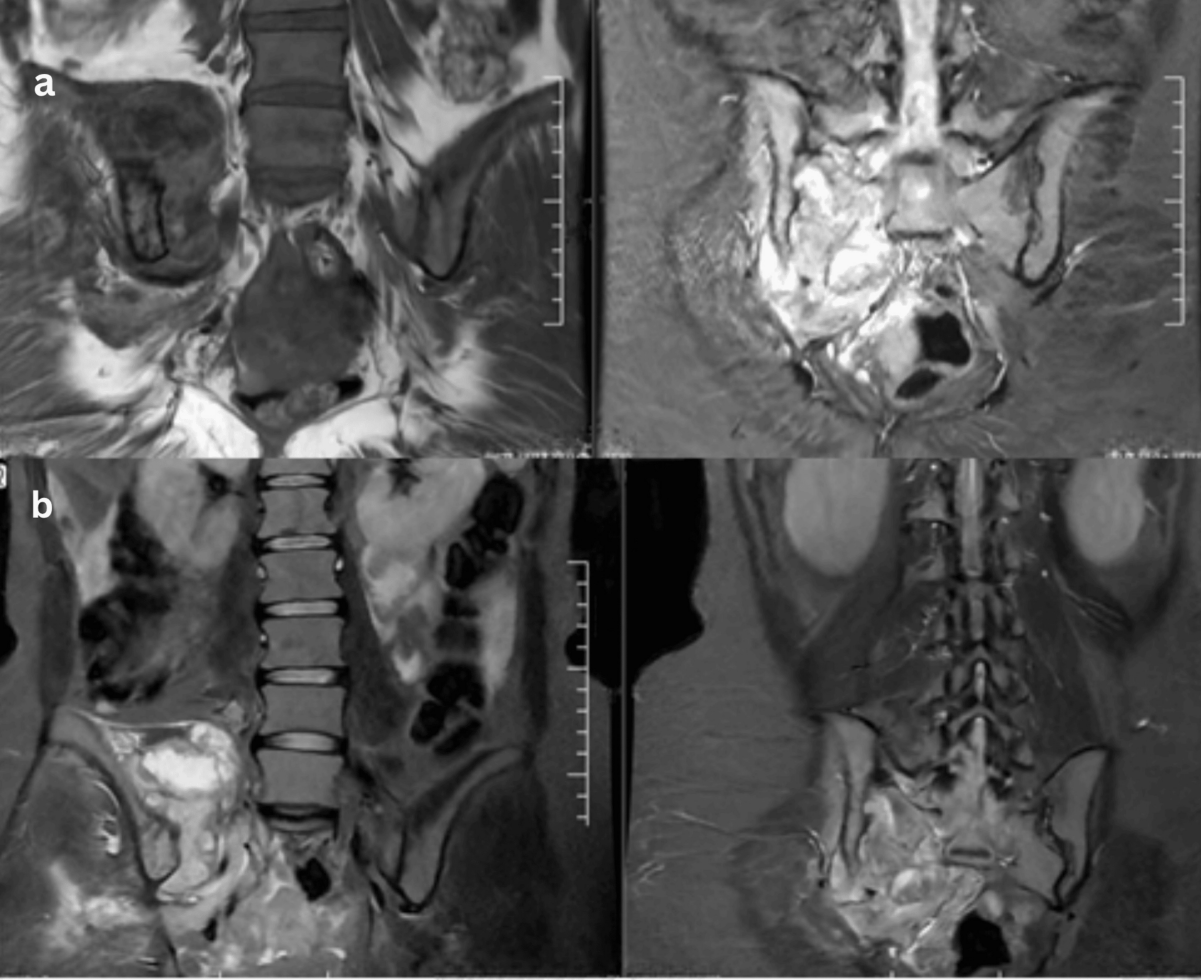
Spinal case 14 (a, b) Plain MRI and Contrast MRI, respectively, of the lumbosacral spine shows extensive bone marrow edema in the juxta articular regions of the right iliac bone and sacrum, further extending into the right sacral ala with evidence of bone abscess, significant synovial effusion, and multiple periarticular, peri-osseous, and intramuscular abscesses noted around the right sacroiliac joint. There is further extension of infection/abscesses into bilateral sacral neural foramina and lumbosacral spinal canal causing severe compression over cauda roots and severe spinal canal stenosis. Data shown are representative MRI findings from a single patient (spinal case 14). Original images obtained by the authors at Subbaiah Institute of Medical Sciences, Shivamogga.
